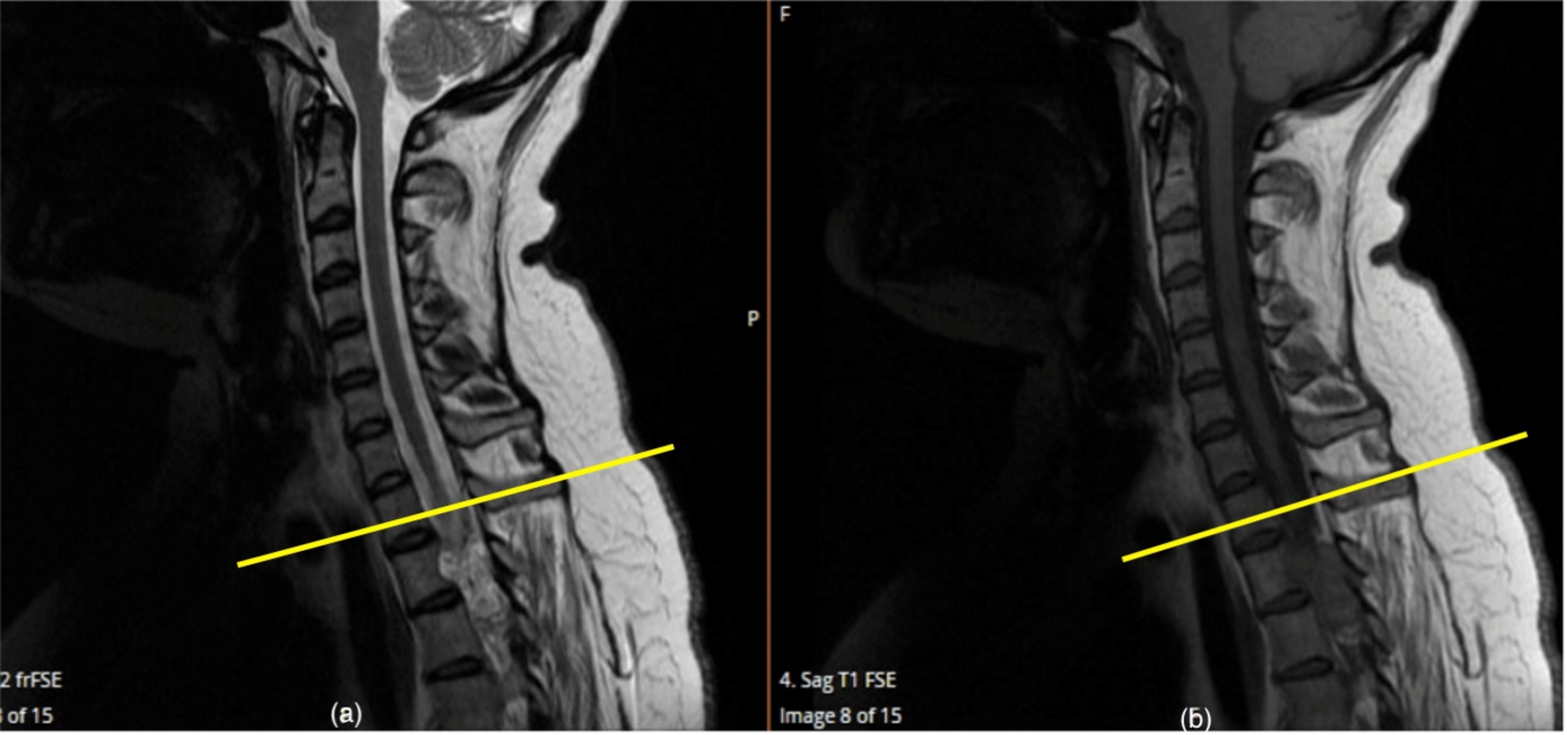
T2 sequenced and T1 sequenced MRI images (a) T2 sequenced and (b) T1 sequenced MRI of the sagittal section of the whole spine showing signs of cavernoma extending inferiorly to T2/T3 exit foramen - likely causing compression of T2 nerve root (marked below the yellow arrow lines) MRI: Magnetic resonance imaging
